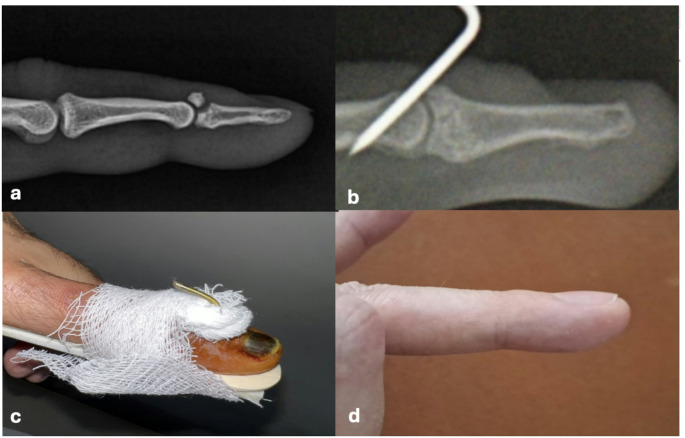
The pin orthosis-extension block pinning technique. a Doyle-type 4b mallet fracture lateral radiography image. b Postoperative lateral radiography image. c Sagittal plane clinical image after orthosis placement. d Clinical image at final follow-up demonstrating functional outcome
